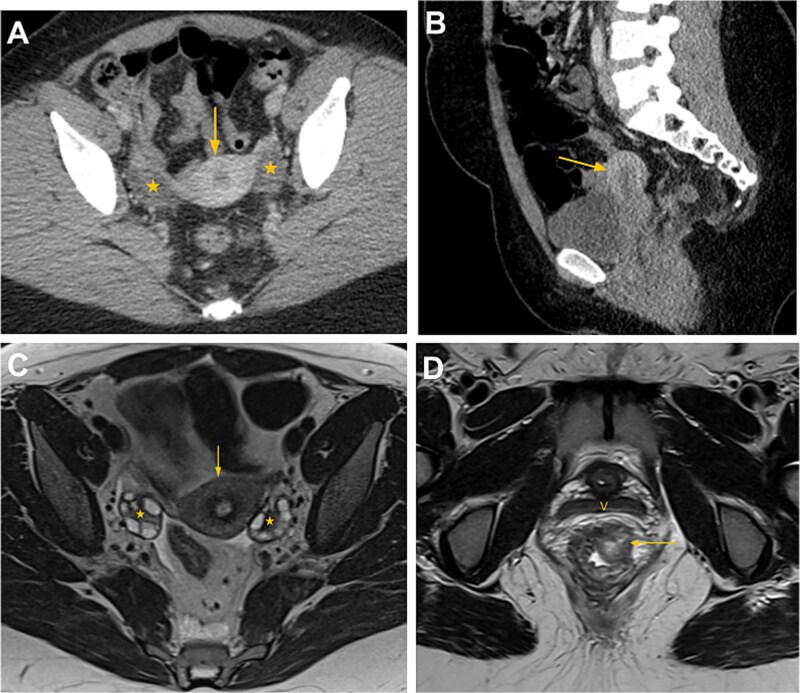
Computed tomography (CT) and magnetic resonance imaging (MRI) of the pelvis prior to uterine transplantation. Axial (A) and sagittal (B) contrast-enhanced CT images of the pelvis demonstrate the uterus (arrow) and bilateral ovaries (*) in normal position. (C) Axial T2-weighted MRI image shows normal pelvic anatomy with the uterus (arrow) and ovaries (*) containing physiological follicles. (D) Axial T2-weighted MRI image at the level of the vagina (V) demonstrates a 1.8 × 2.1 cm polypoid, heterogeneous lesion involving the left anterolateral aspect of the distal rectal wall, consistent with adenocarcinoma (arrow).
